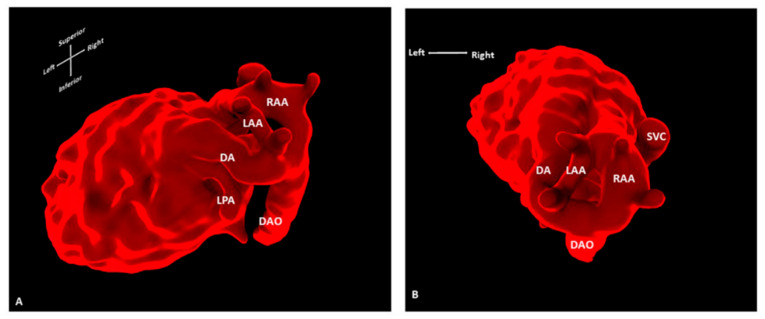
Three-dimensional foetal cardiac MRI showing a double aortic arch. Segmentation of motion-corrected 3D foetal cardiac MRI data, showing (A) superior and (B) oblique left lateral/superior view of the foetal heart. Foetal cardiac MRI was conducted at 32 + 4 weeks’ gestation for suspected double aortic arch. Surgical findings confirmed the presence of a smaller left aortic arch in addition to dominant right aortic arch and left arterial duct. AD: Arterial duct; DAO: Descending aorta; LAA: Left aortic arch; LPA: Left pulmonary artery; RAA: Right aortic arch; SVC: Superior vena cava.
